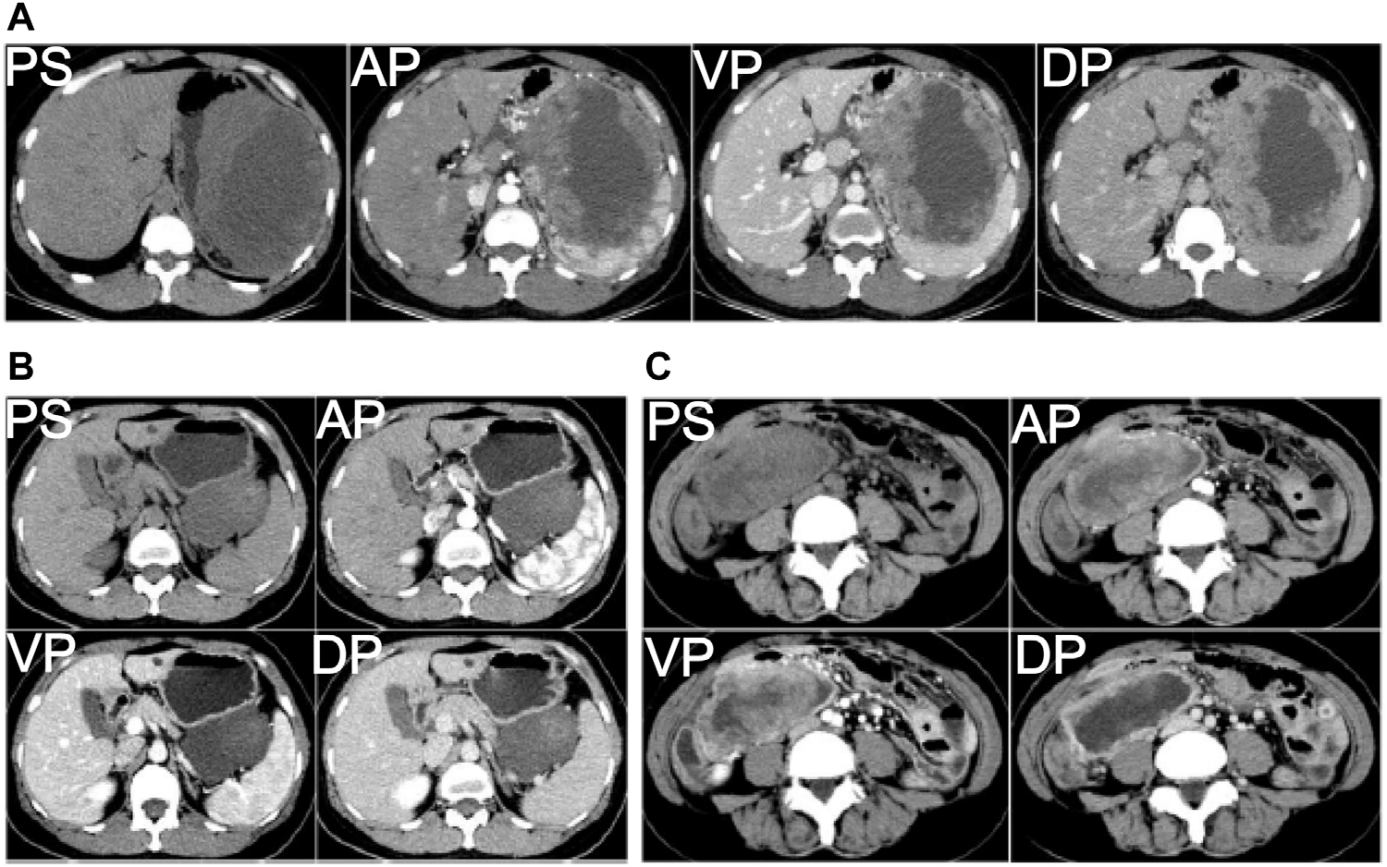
CT imaging of the female patient at different phases of the tumor progression. (A) CT imaging of the female patient at the initial diagnosis in the Xinxiang First People’s Hospital (photo taken on 2017-08-06). (B) Primary tumor shrinking after imatinib treatment for around four and a half months (photo taken on 2017-12-27). (C) Recurrent tumor was observed seven and a half months after primary tumor resection (photo taken on 2018-08-13). PS: plain scanning, AP: arterial phase, VP: venous phase, DP: delay phase.
